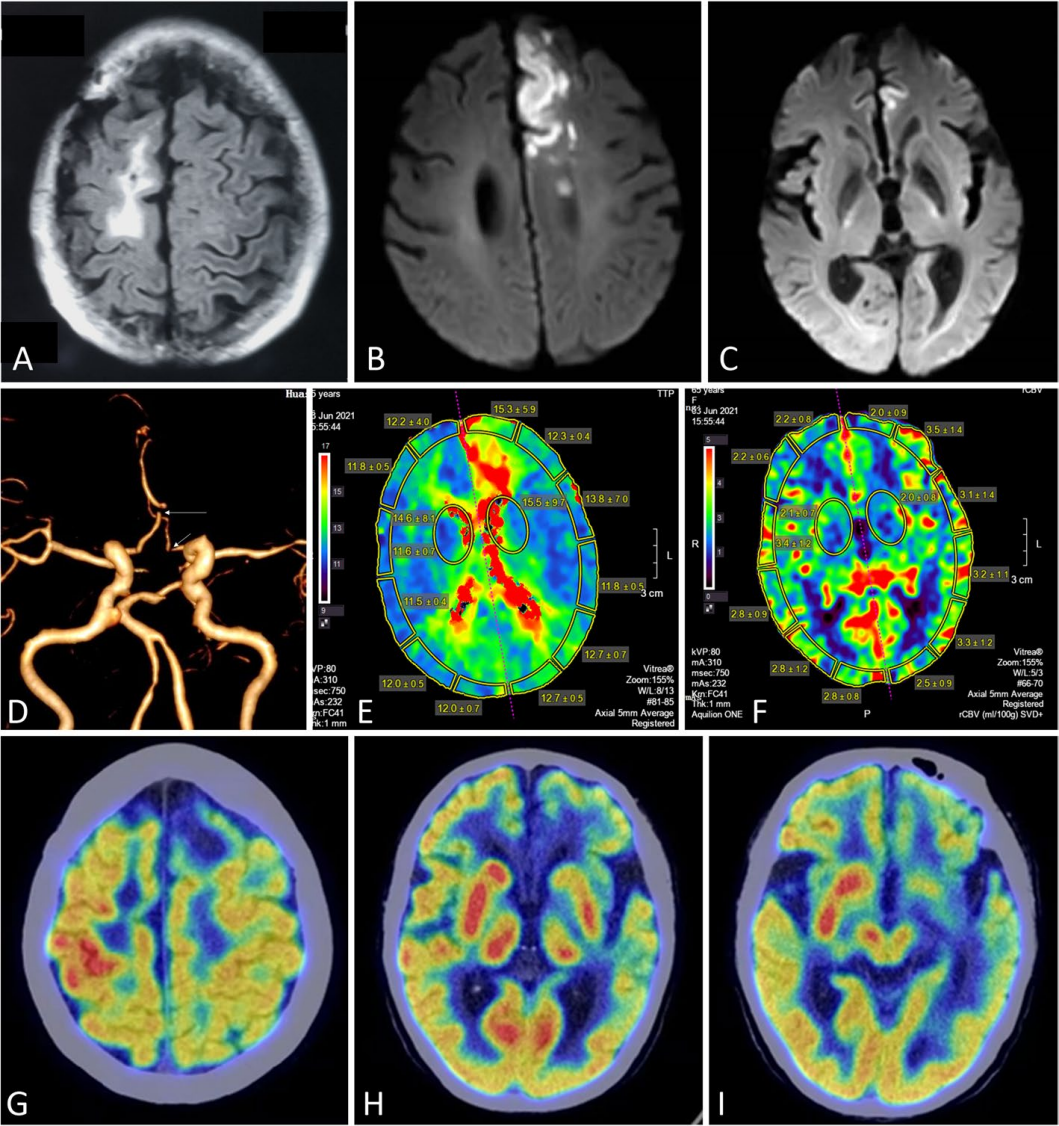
A. Fluid attenuated inversion recovery sequence showed an old lesion in the left frontal lobe 9 days before admission. B and C. Diffusion weighted imagines demonstrated acute left frontal lobe infarction without basal ganglia region involvement after admission. D. CT angiography revealed stenosis in both anterior cerebral arteries (arrows). E and F. CT perfusion revealed delayed perfusion in the left frontal cortex, as shown in the time-to-peak (TTP) with normal perfusion of deep structures. G, H and I. 18FDG PET-CT showed hypometabolism in the left frontal lobe and hypermetabolism in the right central sulcus and basal ganglia region
